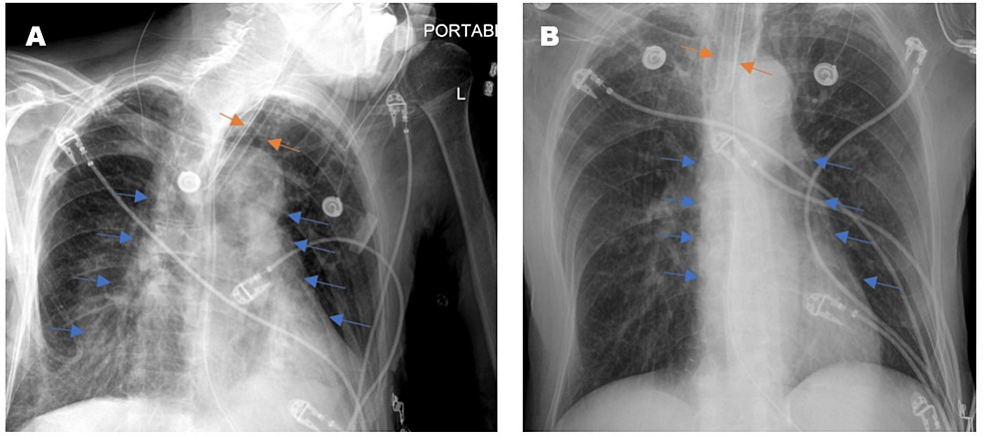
Two PCXRs from a single floor patient taken at different times of the same day. The rotated film (A) grossly exaggerates the cardiomediastinal silhouette (blue arrows) and alters the appearance of endotracheal tube positioning (orange arrows). PCXRs: portable chest x-rays
